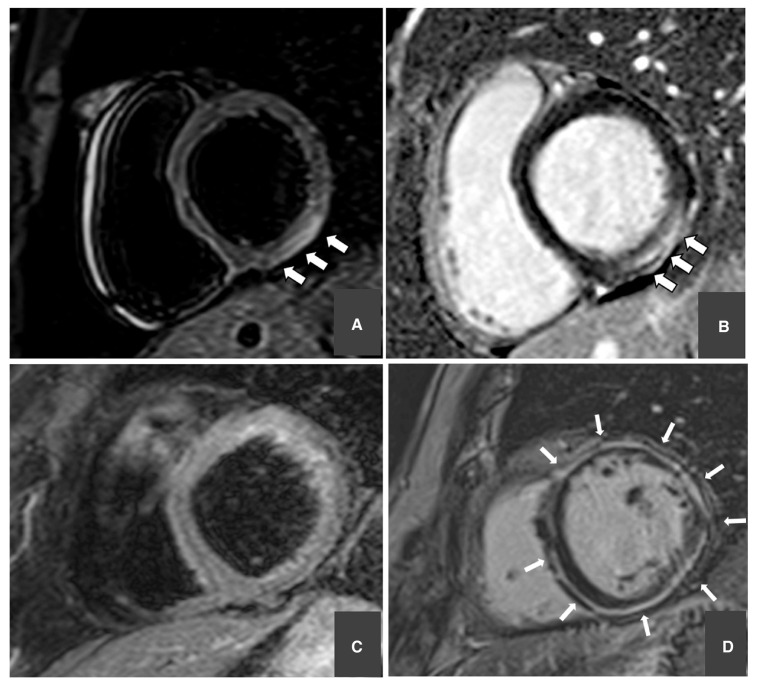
Cardiac magnetic resonance (CMR) T2-weighted (left) and late gadolinium enhancement (LGE) sequences (right). Short axis views showing subepicardial myocardial edema of the inferolateral left ventricular wall ((A) arrows) and subepicardial late gadolinium enhancement (LGE) in the same segment ((B) arrows) in a patient with normal coronary artery, suggesting acute myocarditis. Absence of myocardial edema (C) with circumferential subepicardial-midwall LGE ((D) arrows) in a patient with left-dominant arrhythmogenic cardiomyopathy. Adapted with kind permission from Zorzi et al. [34].
